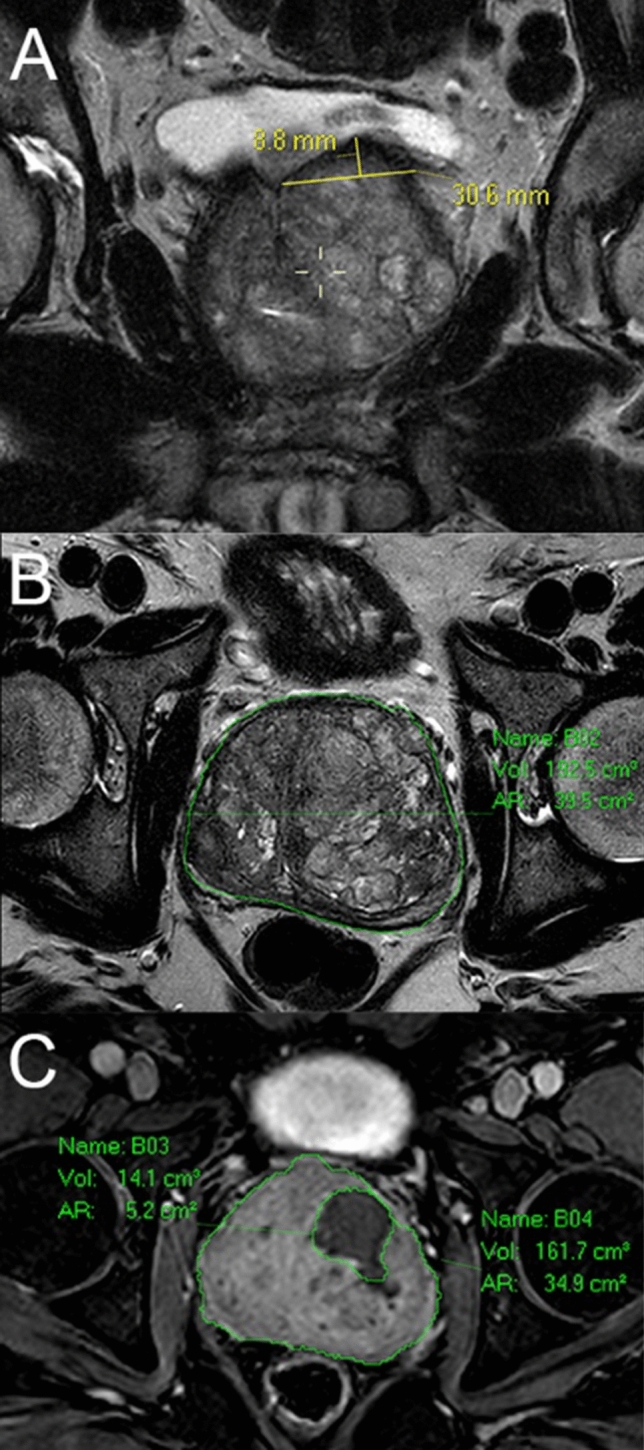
MRI Pre and Post-PAE A – Coronal T2 image of the prostate with the thickness (30.6 mm) and height (8.8 mm) of the intravesical prostatic protrusion measured. The calculated THR would be 3.4 B – Axial T2 image of the prostate with semi-automated volumetry of the gland shown (192.5 ml) C – T1 fat-saturated post-contrast image at 5 min delay showing the total post-PAE PV on the right-hand side (161.7 ml) [constituting a volume reduction by 16%] and the volume of the infarcted portion (14.1 ml) shown on the left-hand side. This constitutes an infarction percentage of 8.6%. MRI – magnetic resonance imaging, PAE – prostate artery embolization, T1 – Time 1 weighted, T2 – Time 2 weighted, THR – thickness-to-height ratio
